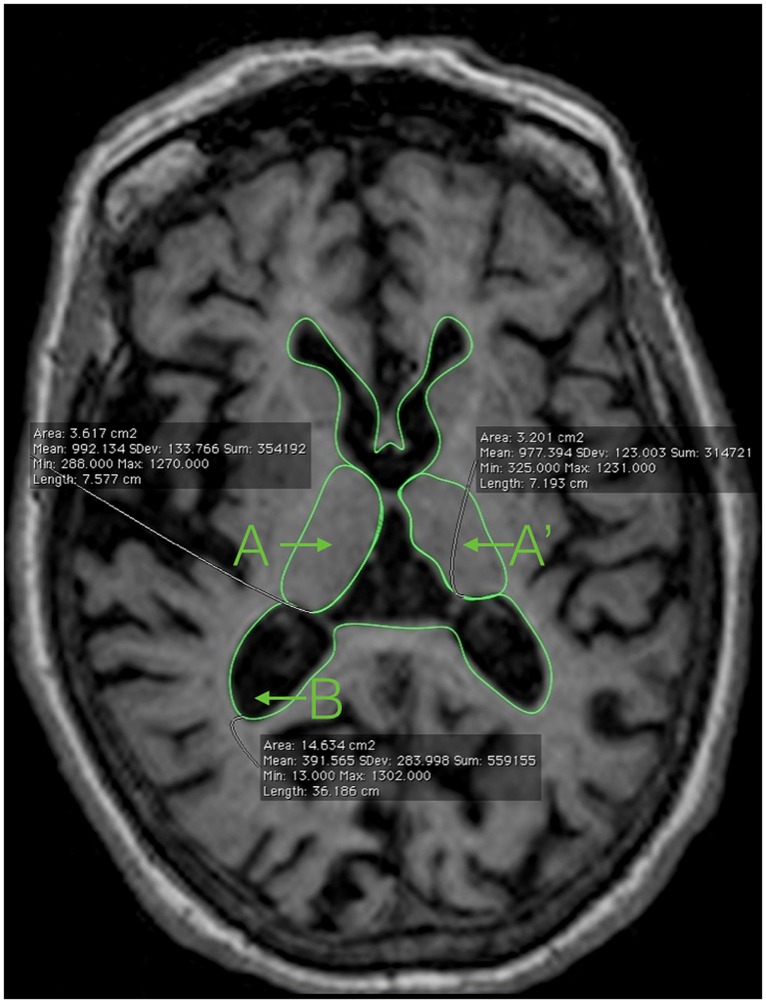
Magnetic resonance imaging showing the areas needed to compute the 2D-yrRTA: A and A’: right and left thalamus; B: ventricular system including the lateral ventricles and the third ventricle.
